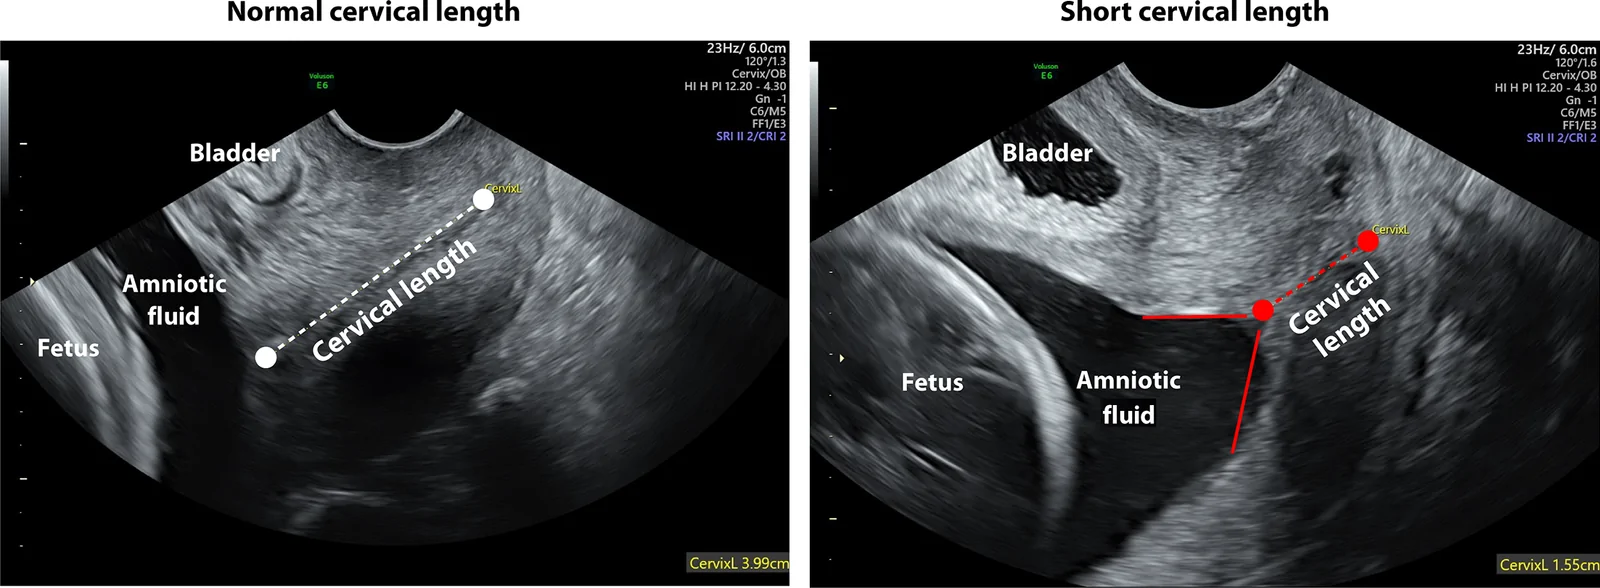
A transvaginal ultrasound demonstrates the measurement of cervical length. A normal cervical length (≥2.5 cm) with a T-shape cervical length (left). A short cervix shows cervical length of <2.5 cm with Y-shaped funneling (right).
